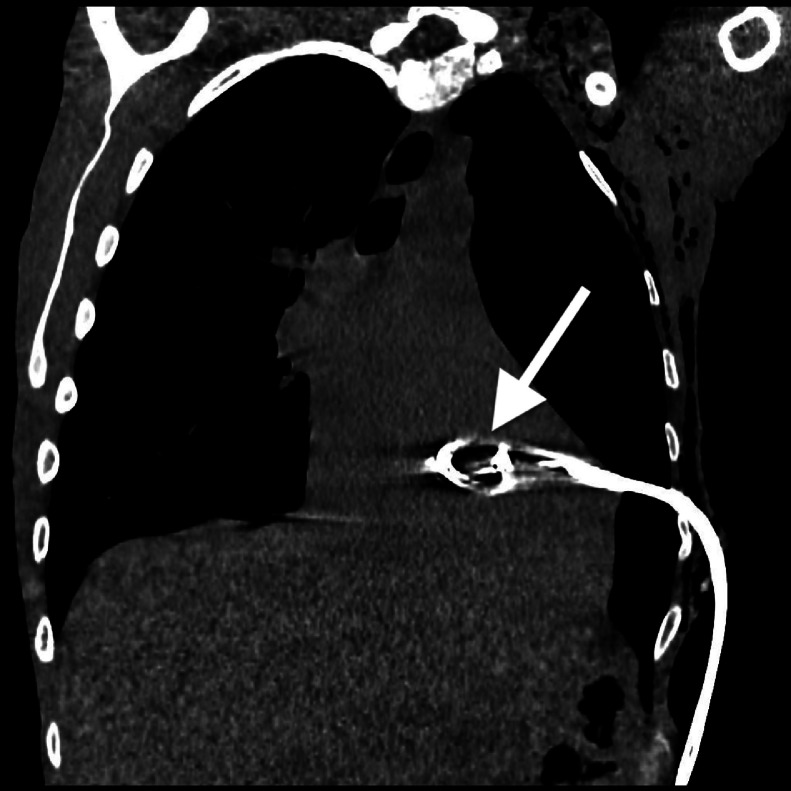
Coronal CT image of the chest (mediastinal window) showing iatrogenic intracardiac chest tube placement (white arrow).
